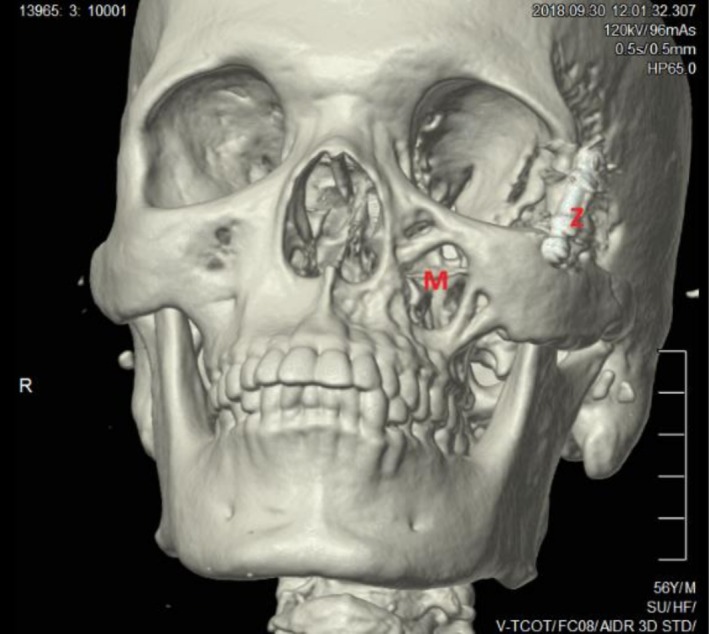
Facial skeleton computed tomography (CT) scan shows destruction of bone involving the (Z) zygomatic arch and the (M) anterior surface of the left maxillary antrum.
